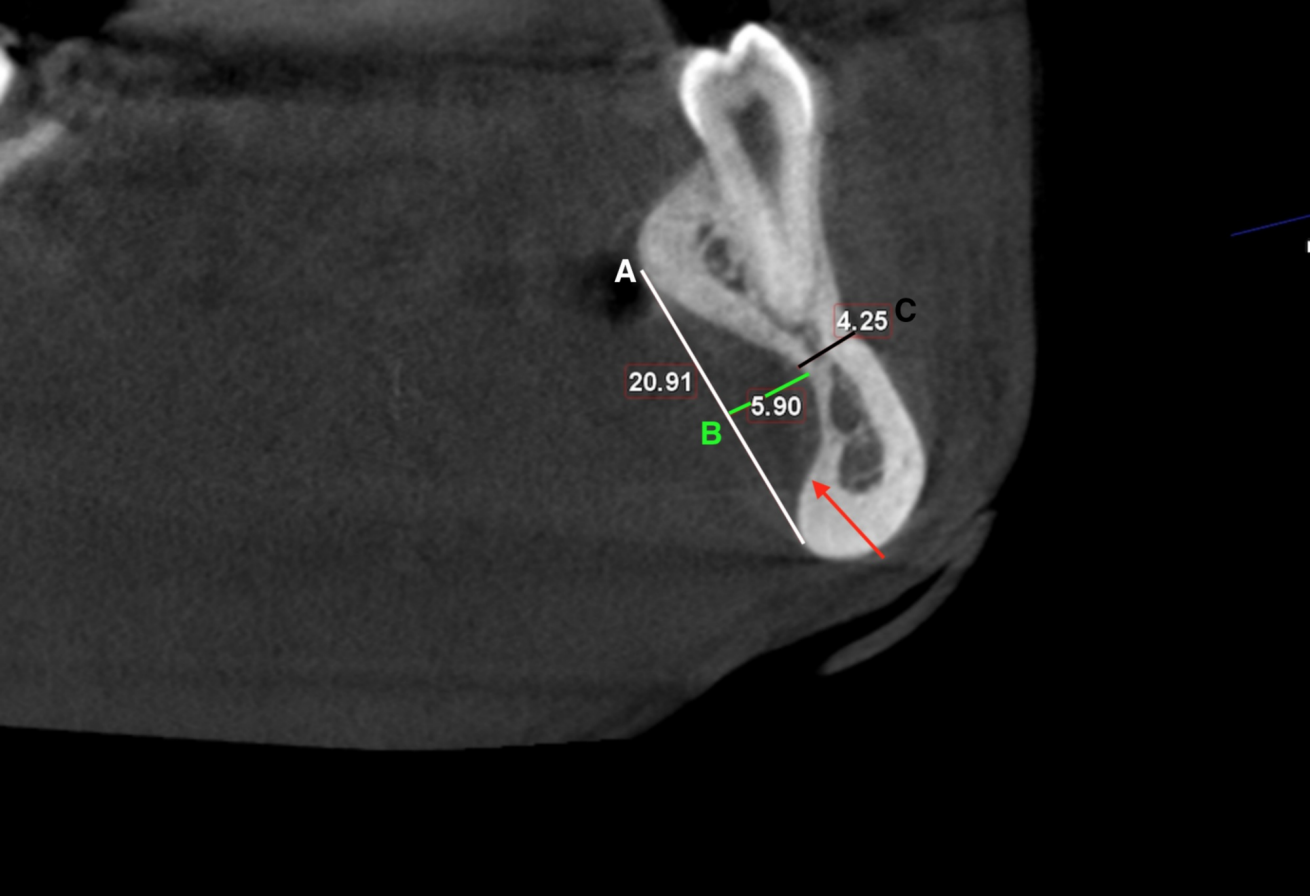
Cross-sectional cone beam computed tomography of the left lingual depression Lingual depression (red arrow), a line tangent to the heights of contours of the lingual surfaces of the mandible (A), the deepest point of the depression (B), the buccolingual thickness of the remaining mandible (C).
